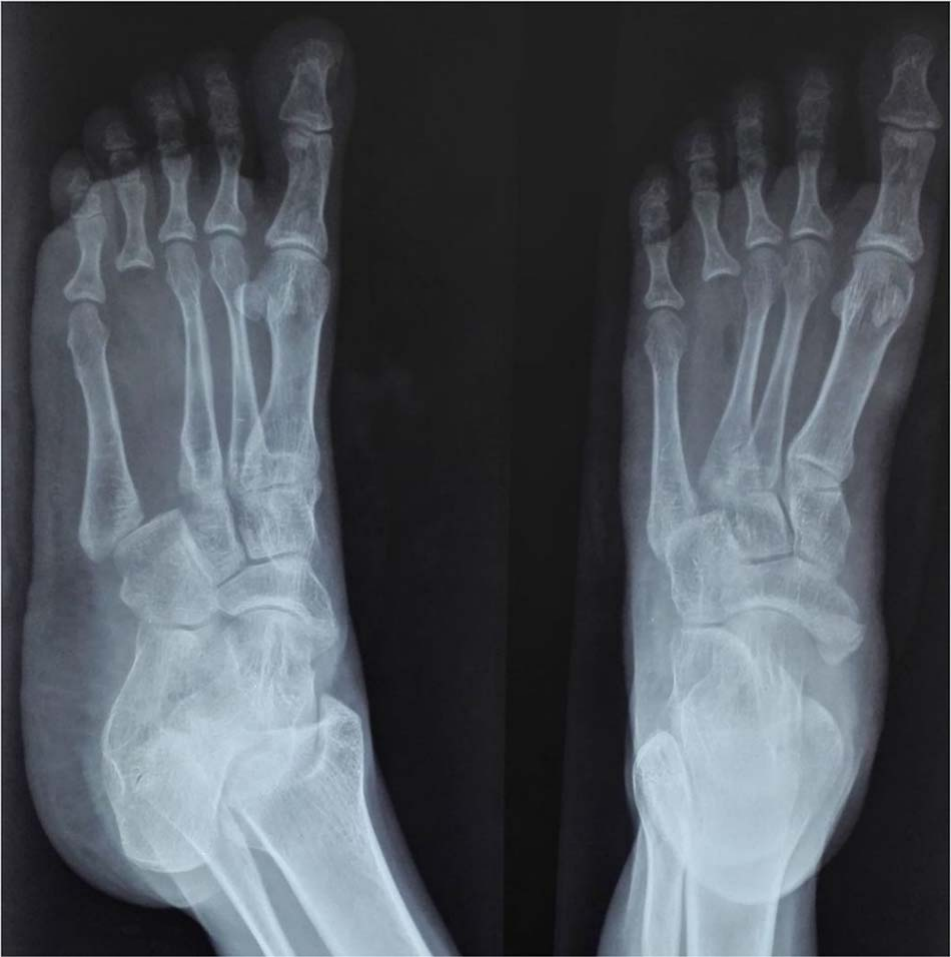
Post-operative radiograph showing preserved joint space and structure of transferred metatarsal.
